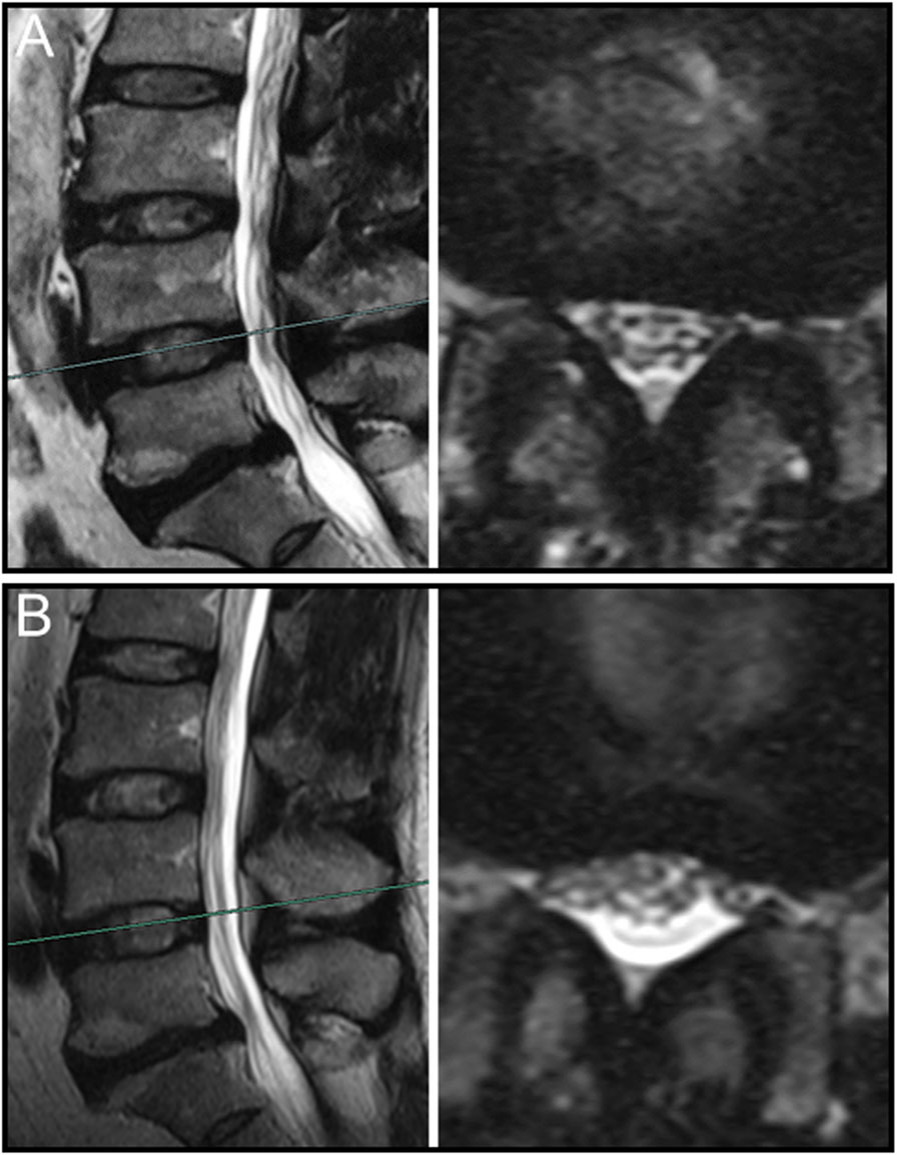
In a woman with positive SedSign (a), the nerve roots subsided to the ventral side of dural sac on prone MR images (b)
